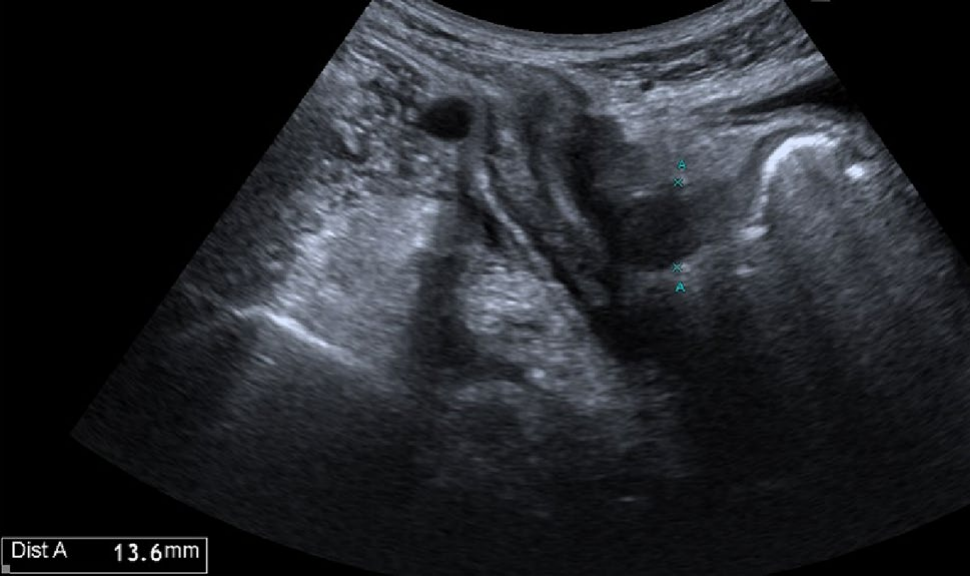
In the right iliac fossa, the final loop of the ileum shows, over a segment of 7 cm, thickened walls measuring 5 mm, with preserved stratification and the presence of vascular signals in the walls as detected by color Doppler imaging (Limberg 3). Another segment, located more proximally in the ileum and observed in the hypogastric region, measures approximately 5 cm, with walls thickened up to 10 mm, loss of stratification, and the presence of vascular signals in the walls on color Doppler imaging (Limberg 4). These segments show a narrowed lumen and dilation of the upstream bowel loop (55 mm diameter)
